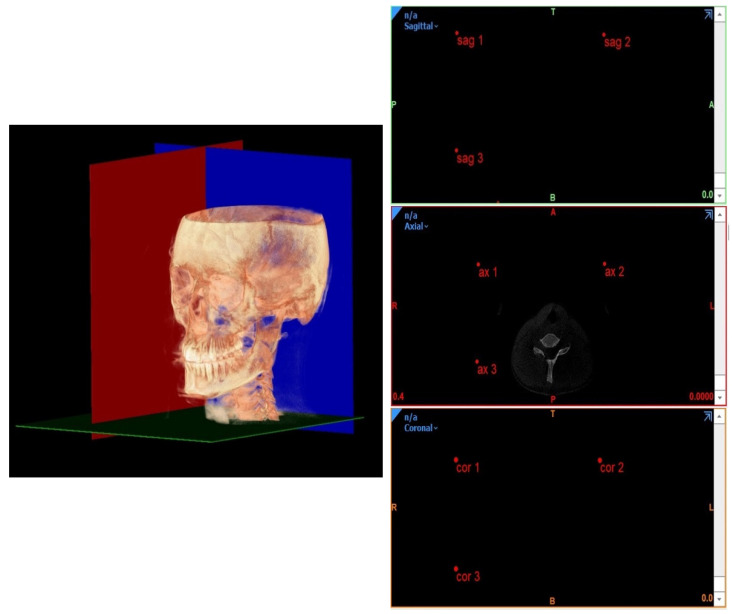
CBCT orientation according to three reference planes identified by selecting 3 points on the most external slice of the CBCT scan, respectively, for every single view (coronal, axial, and sagittal).
